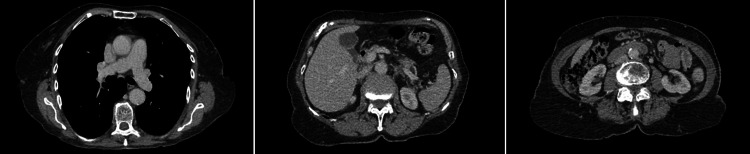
Representative axial CT images of the thorax, abdomen, and pelvis demonstrating no evidence of mediastinal or abdominal lymphadenopathy or mass lesions.
